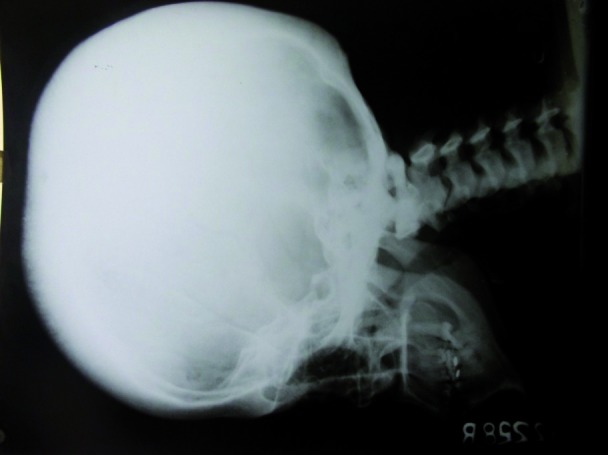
Skull radiograph showed thick and sclerotic skull vault with hypoplastic frontal sinus with mid face hypoplasia.
